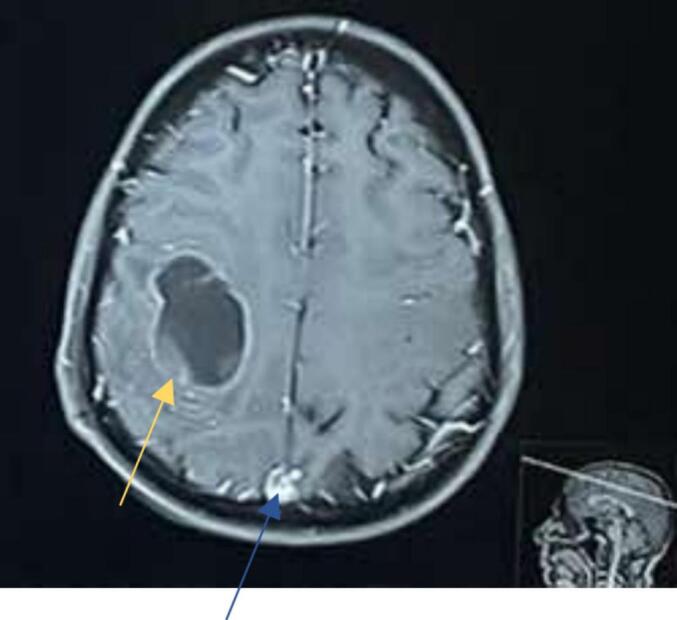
MRI brain with axial section showing Meningioma (blue arrow) and Glioma (yellow arrow). (For interpretation of the references to colour in this figure legend, the reader is referred to the web version of this article.)
